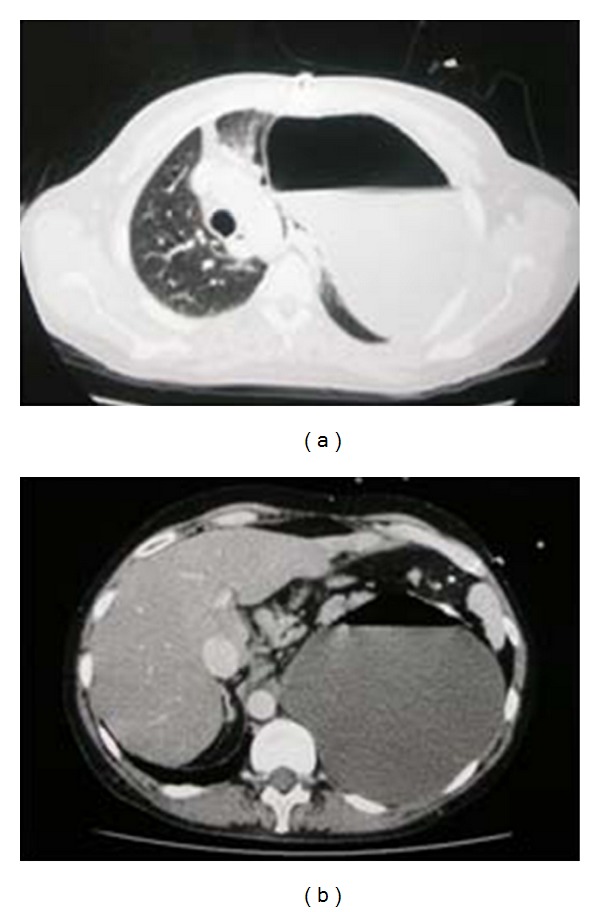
Axial CT scans on the same patient as in Figures 13 and 14 showing diaphragmatic rupture and herniation of the stomach into the left hemithorax.
